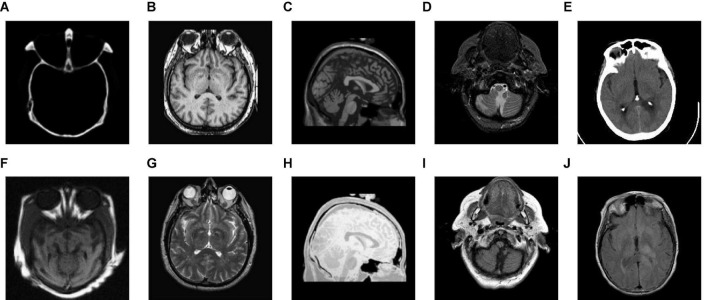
Source image sets for fusion testing. (A–C,E) CT images. (D) MR-T1 image. (F–H) The corresponding MRI images. (I) The corresponding MR-T2 image. (J) Proton density-weighted MR image.
